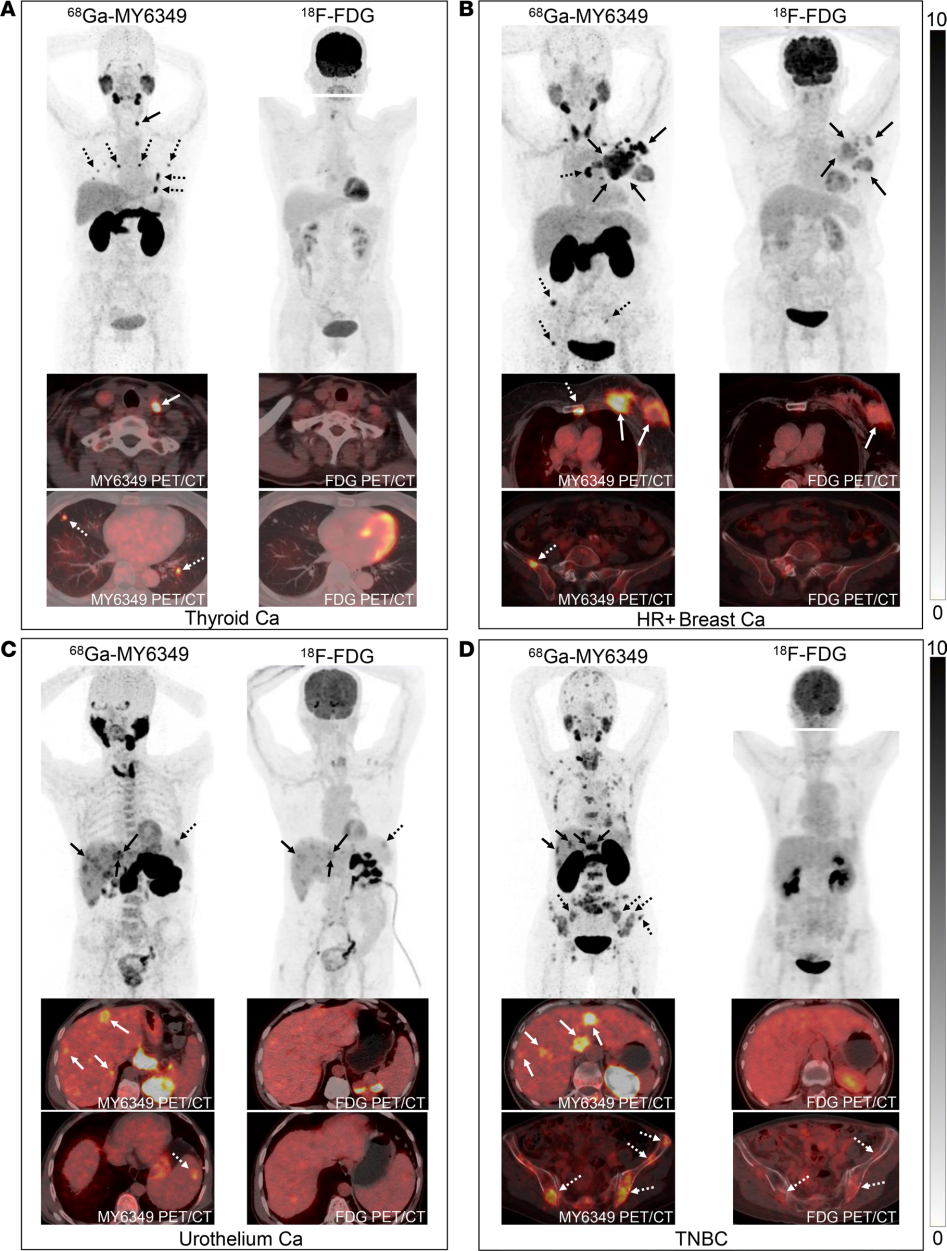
Representative ^18^F-FDG PET/CT and ^68^Ga-MY6349 PET/CT images in patients with various cancers. (A) A 68-year-old woman with papillary thyroid carcinoma had undergone surgical resection 1 year prior; PET/CT was performed to detect tumor recurrence. ^68^Ga-MY6349 PET/CT showed intense uptake in the neck lymph node (solid arrows) and lung metastases (dotted arrows), but ^18^F-FDG PET/CT demonstrated false-negative findings. (B) A 74-year-old woman with HR^+^ breast cancer underwent PET/CT for tumor staging. ^68^Ga-MY6349 PET/CT showed higher radiotracer uptake than that of ^18^F-FDG PET/CT in the primary tumor (solid arrows), lymph node, and bone metastases (dotted arrows). Additionally, ^18^F-FDG PET/CT showed false-negative uptake in the bone metastases. The HR^+^ breast cancer image from Figure 4 is shown again in B. (C) A 63-year-old man with urothelial cancer underwent PET/CT for initial staging. ^68^Ga-MY6349 PET/CT demonstrated higher tracer uptake and better image contrast than ^18^F-FDG PET/CT in the primary and metastatic lesions, particularly for the liver metastases (solid arrows) and spleen metastasis (dotted arrows). The urothelium cancer image from Figure 4 is shown again in C. (D) A 65-year-old woman with previously treated breast cancer (TNBC) underwent PET/CT for restaging. ^68^Ga-MY6349 PET/CT showed intense tracer uptake in the lymph nodes, liver (solid arrows), and widespread bone metastases (dotted arrows). However, ^18^F-FDG PET/CT showed low to mild uptake in most of the metastases.
